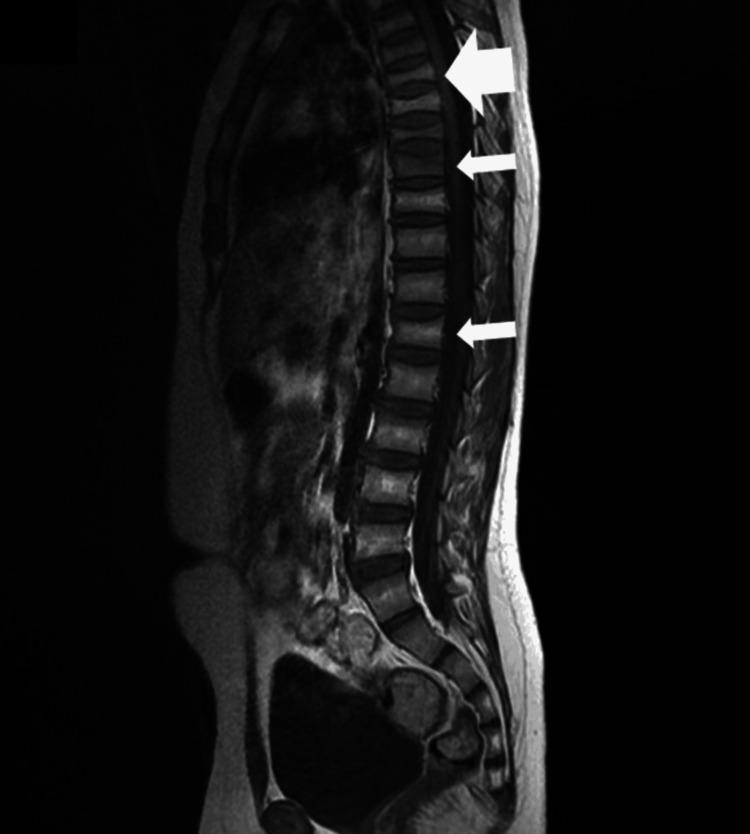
A sagittal T1 contrast-enhanced magnetic resonance imaging demonstrating multiple previous vertebral compression fractures.
